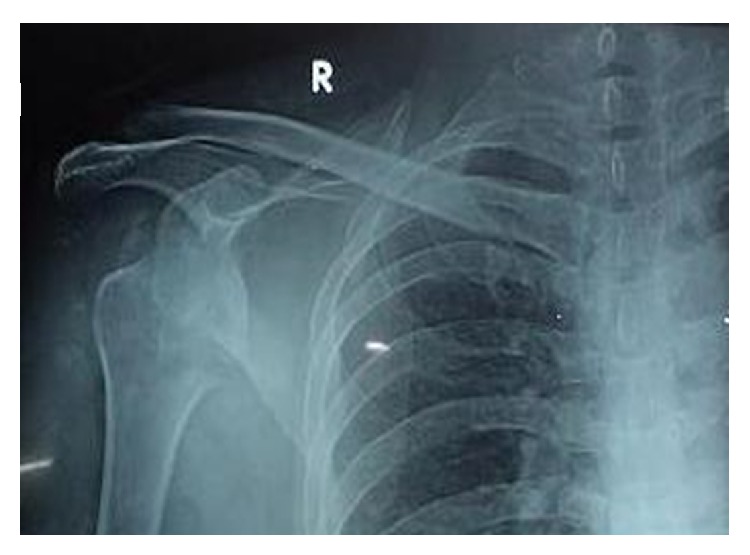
Radiographs of right anterior shoulder dislocation (preoperative).
